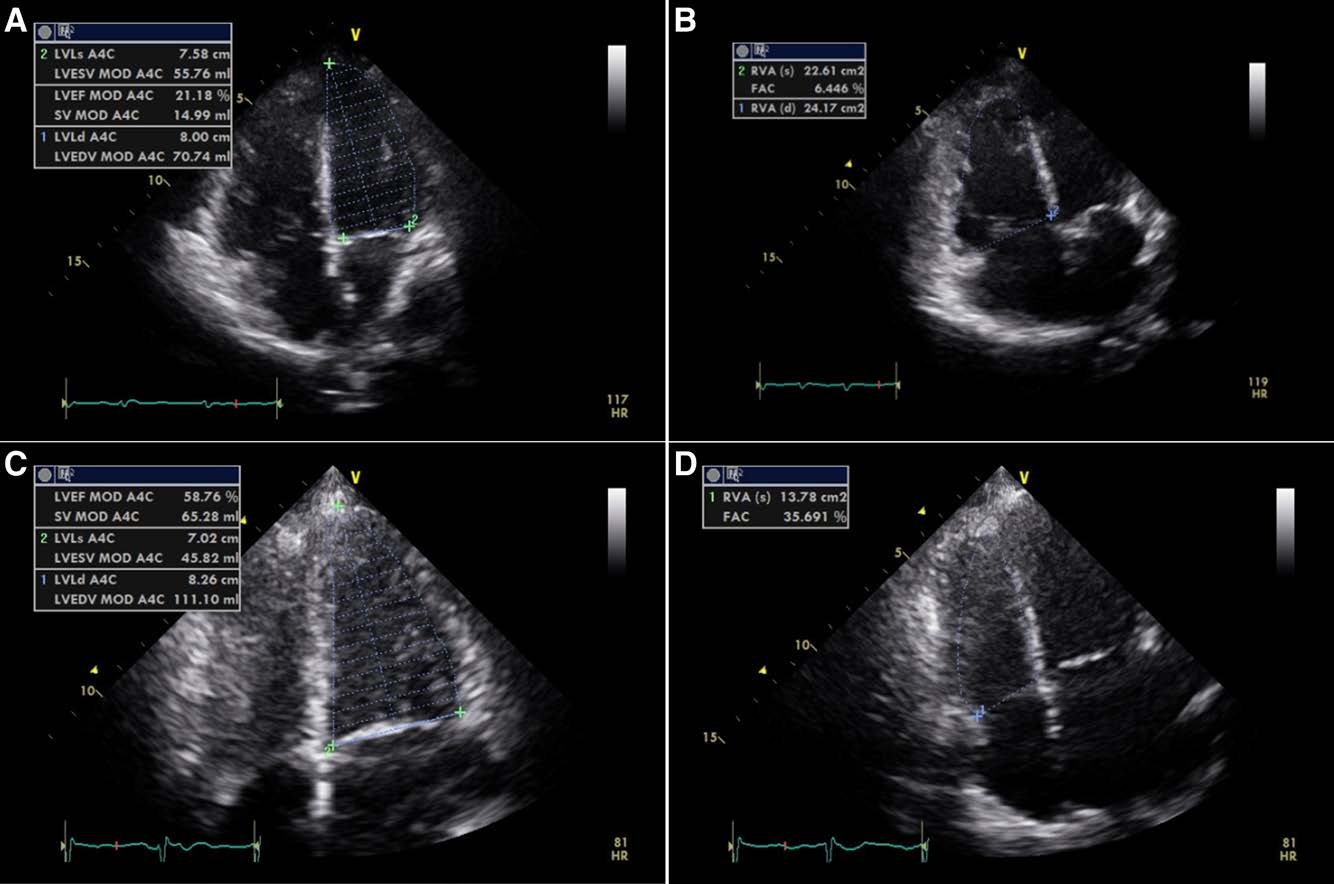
Ejection fraction of left ventricle and changes in fractional area of the right ventricle on the ECMO start and wean date. LV function and RV function of patient by echocardiography on days 2 and 8 of ECMO support. On day 2, RV dysfunction with an FAC of 6% and global hypokinesia was observed to have an overall decrease in LV function with an EF of 28%. On day 8 after ECMO support, the LV function improved (EF 60%), and the initially observed decrease in RV contractility also showed improvement (FAC 35%) in the F/U echocardiogram. ECMO = extracorporal membrane oxygenation, EF = ejection fraction, FAC = fractional area change, LV = left ventricular, RV = right ventricular.
